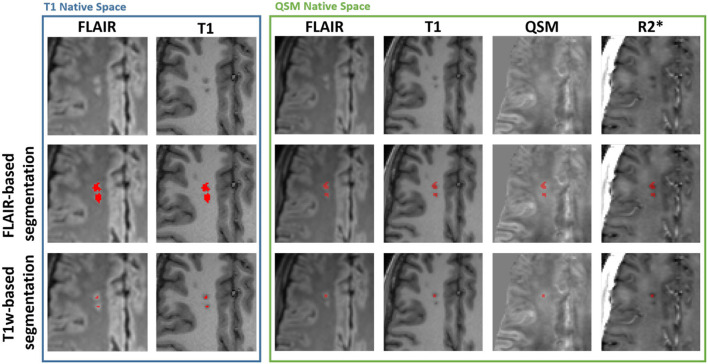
Example silent cerebral infarct segmentations. Blue Box: Axial view of two silent cerebral infarcts (SCI) segmented in a representative patient with sickle cell anemia (SCA) overlaid on FLAIR and T1-weighted images in the native T1w space. The example SCI appear hyperintense on FLAIR images and hypointense on T1-weighted images. The application of a more stringent T1w-based maximum intensity threshold (bottom) reduces the volume of the segmented SCI lesions relative to the standard FLAIR-based segmentation (middle). Green Box: Axial view of the same SCI after interpolation into the native quantitative susceptibility mapping (QSM) space, overlaid over FLAIR, T1w, QSM and R2* images. The SCI appear bright on QSM and dark on R2* maps relative to normal appearing white matter. Note that the coregistration of the lesions into the QSM space results in the lower SCI segmentation appearing in the adjacent slice.
